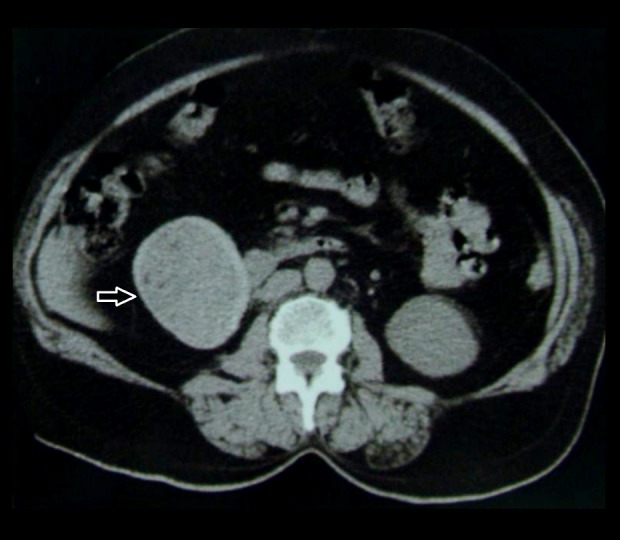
Enhanced CT scan shows a thick-walled structure with high-attenuation contents in the retroperitoneum on the right side.
